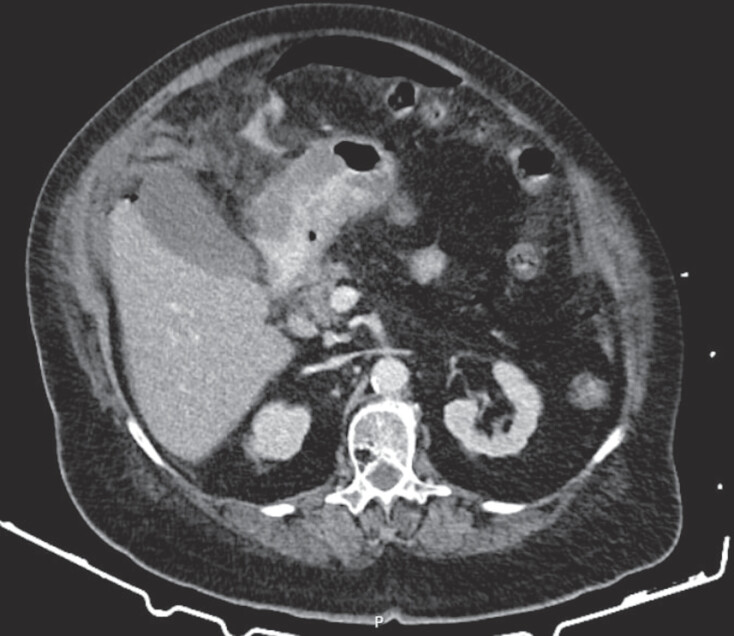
An abdominal computed tomographic scan showing a prepyloric
